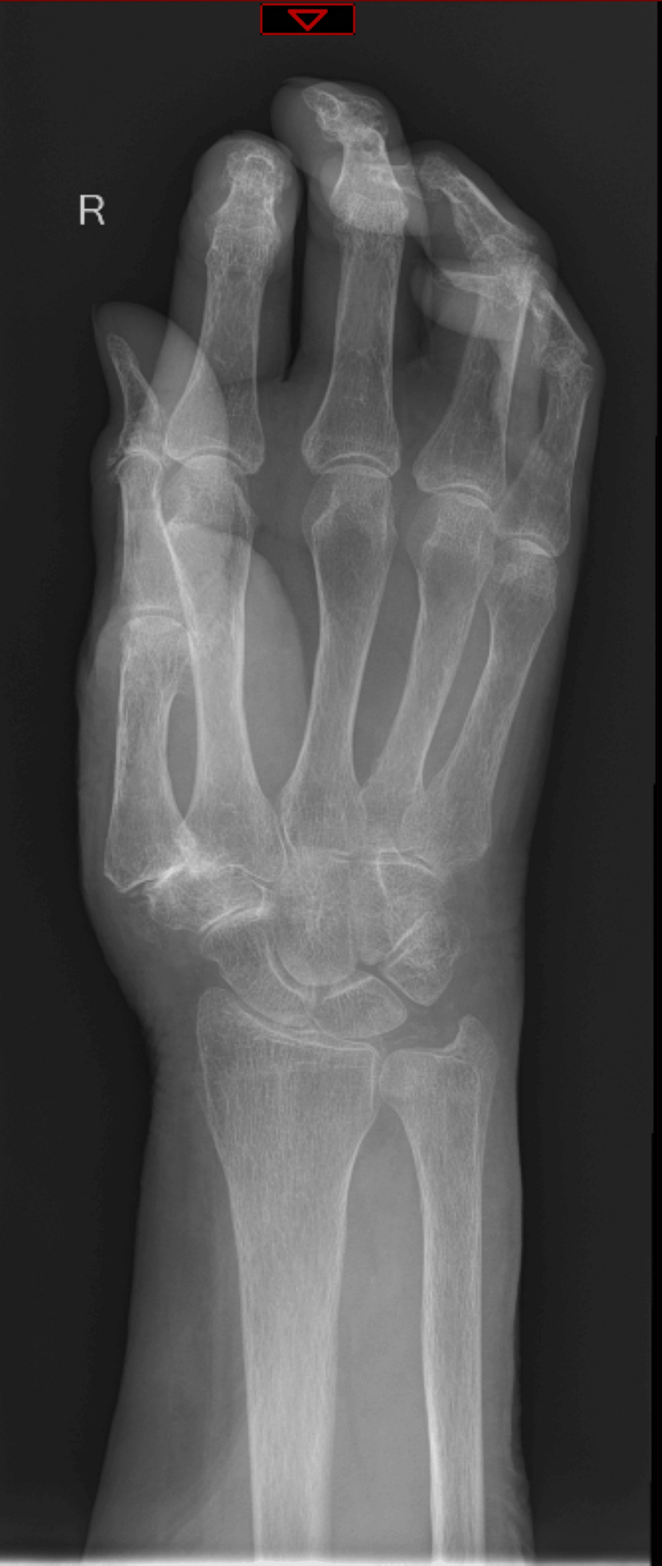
Patient's right hand X-ray and right wrist X-ray showing generalised osteopenia, severe degenerative change at the first carpometacarpal joint and several interphalangeal joints, with no plain film evidence of osteomyelitis
